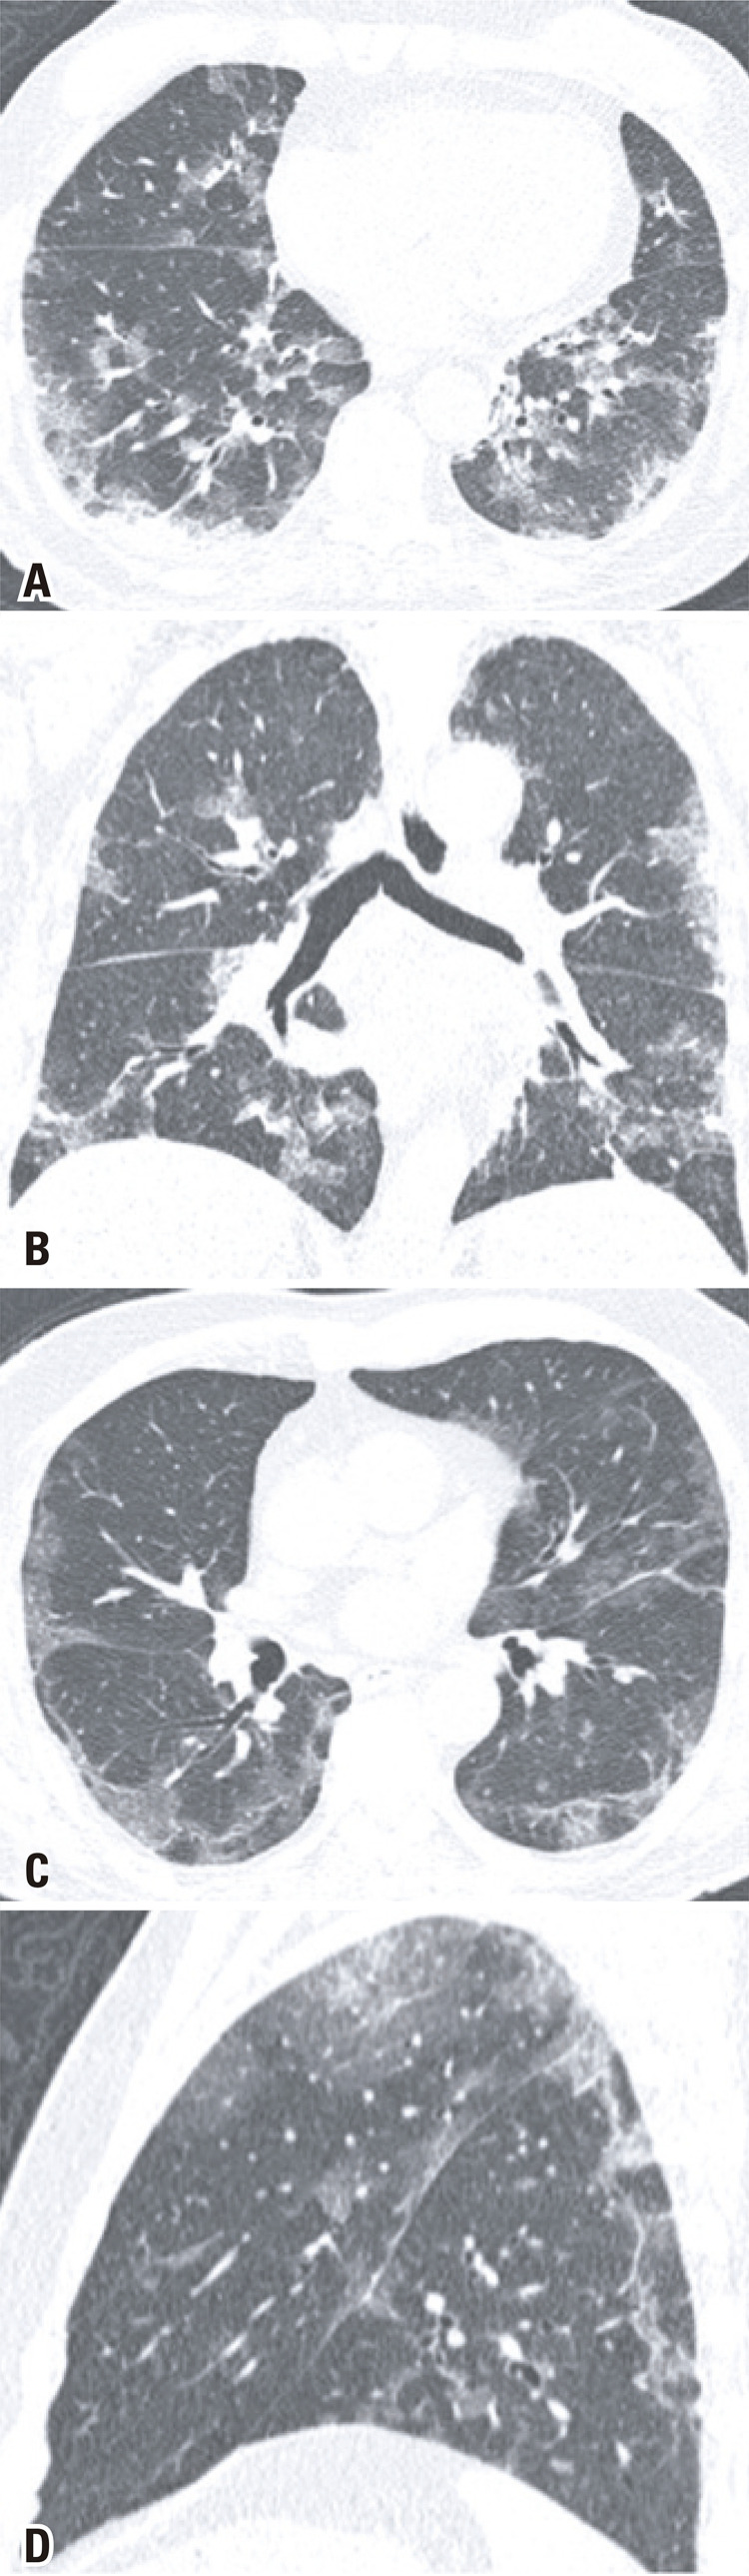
Axial (A, C), coronal (B) and sagital (D) images of chest computed tomography showing typical COVID-19 pulmonary findings
